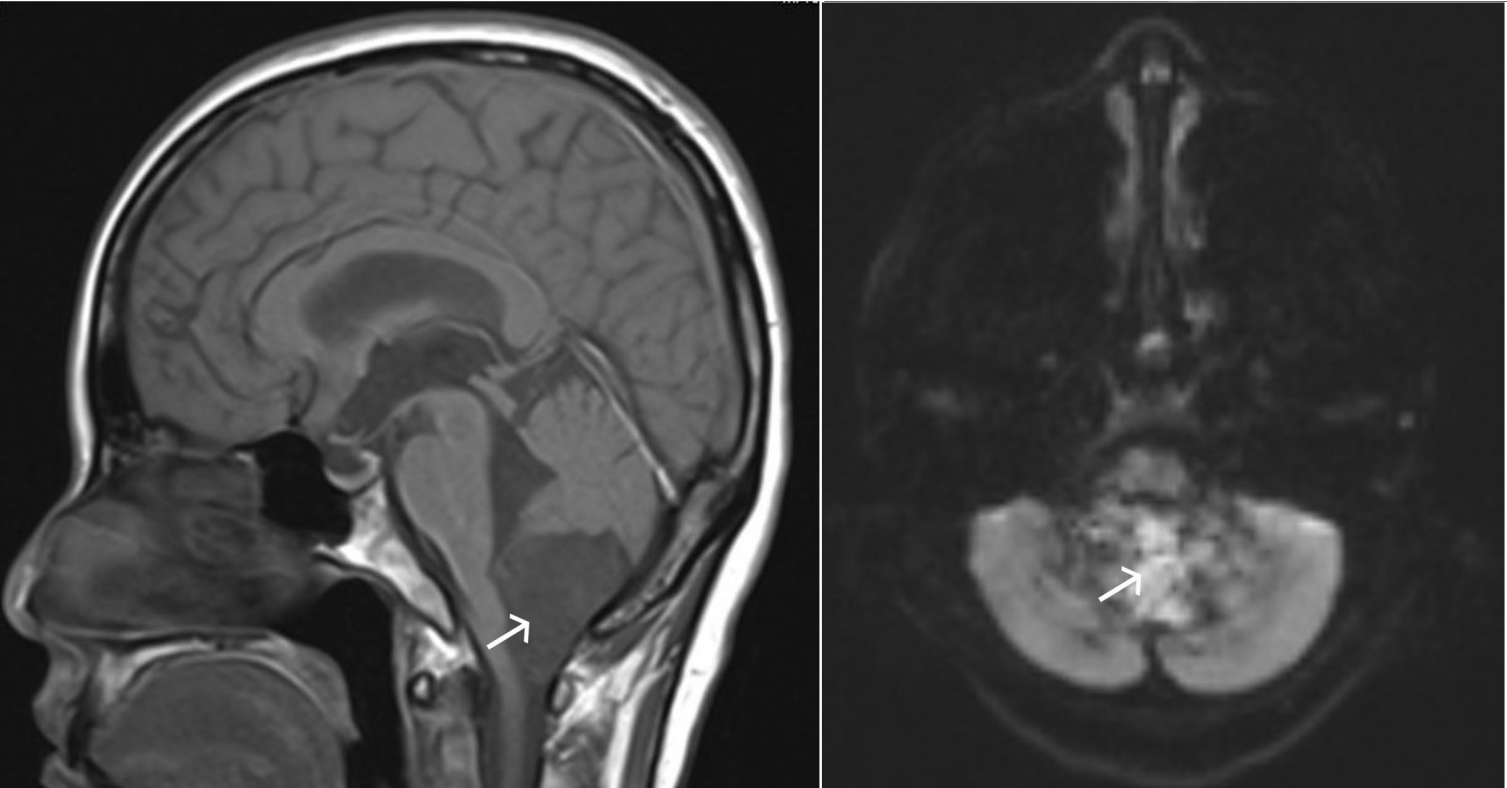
Sagittal T1, axial T2W, and axial DWI MRI of the posterior fossa and cervical spine show a cyst at the craniovertebral junction (white arrow).
